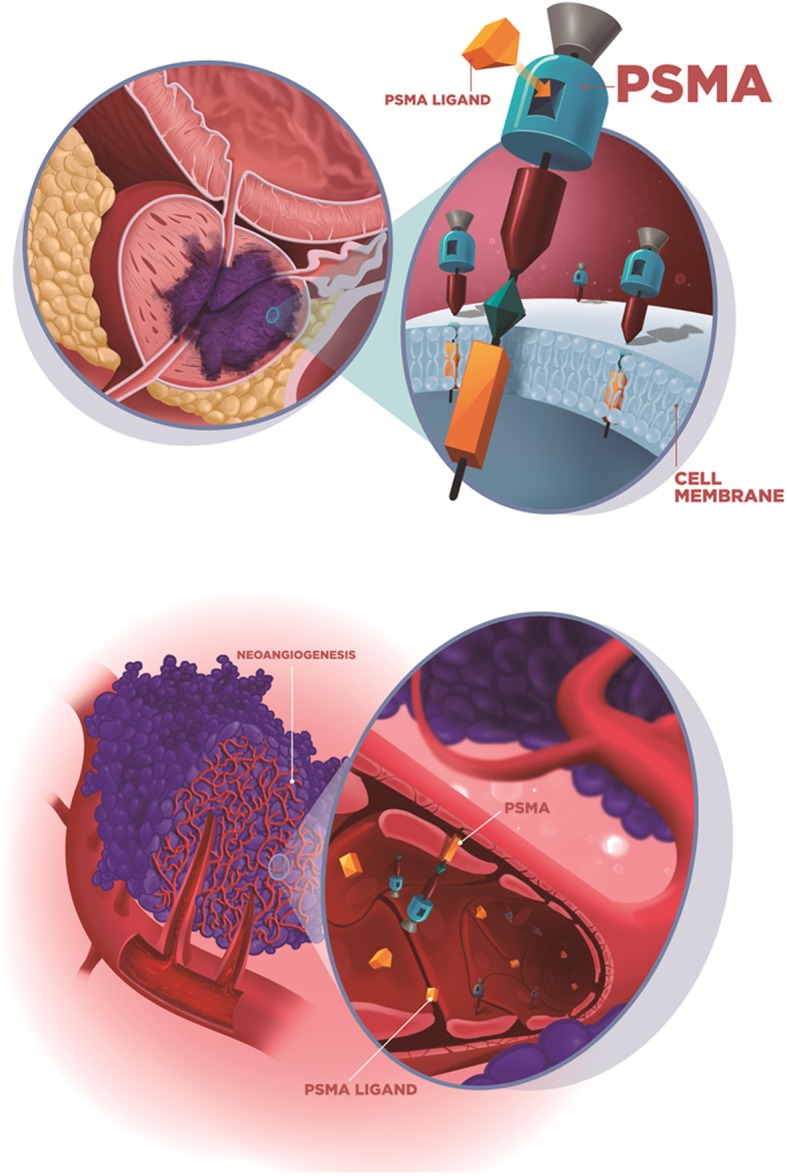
Ilustration of the two main mechanism os PSMA expression that explains the radiotracer uptake in prostate cancer (a) and other non-prostatic causes with endothelial expression (b)
